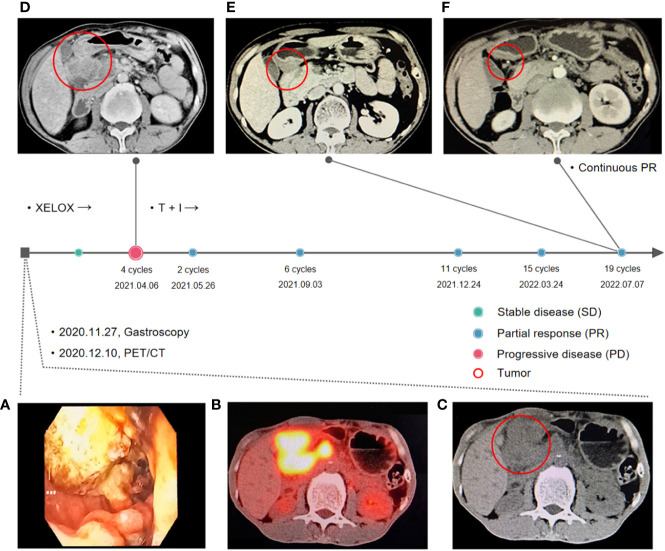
Treatment timeline. (A) Gastroscopy report at first visit: A large annular mass was observed in the duodenal bulb, extending to the upper part of the descending part. (B, C) Positron emission tomography/computed tomography (PET/CT) before the first-line treatment: a significant uneven thickening of the intestinal wall of duodenal bulb (47 × 40 mm) and multiple lymph node metastases. (D) Computed tomography after four sessions of XELOX treatment. The tumor progressed and fused with surrounding lymph nodes after four cycles of XELOX (65 × 63 mm). (E, F) Computed tomography after two and 19 sessions of tislelizumab in combination with irinotecan.
